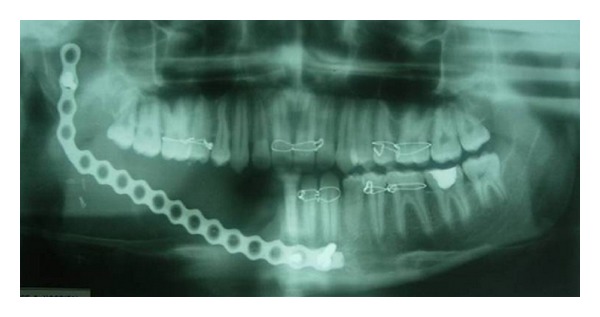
Postoperative panoramic radiograph showing reconstruction of the resected site with microvascular iliac bone grafting and fixation with titanium plate.
